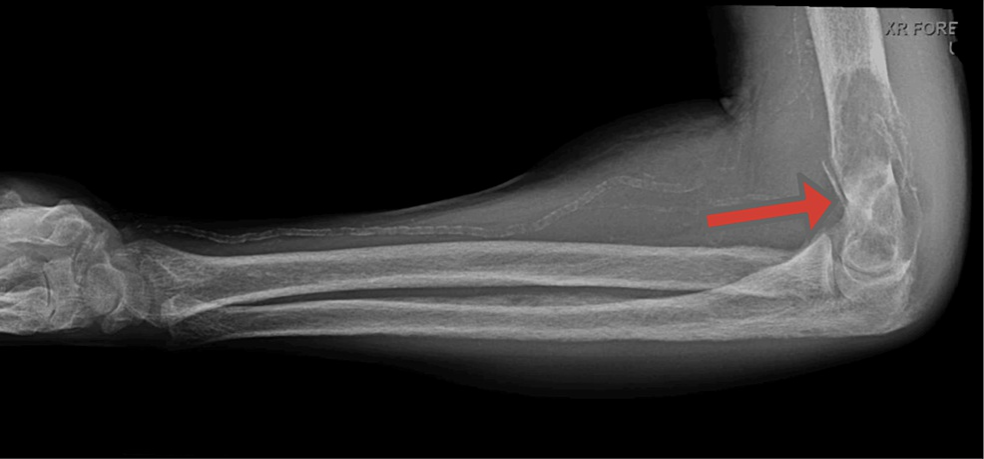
Two-view right forearm X-ray: 8 cm destructive process in the distal meta-diaphysis of the humerus with pathologic fracture, cortical-based focus on the proximal ulna, olecranon enthesopathy, and advanced vascular calcification (arrow)
